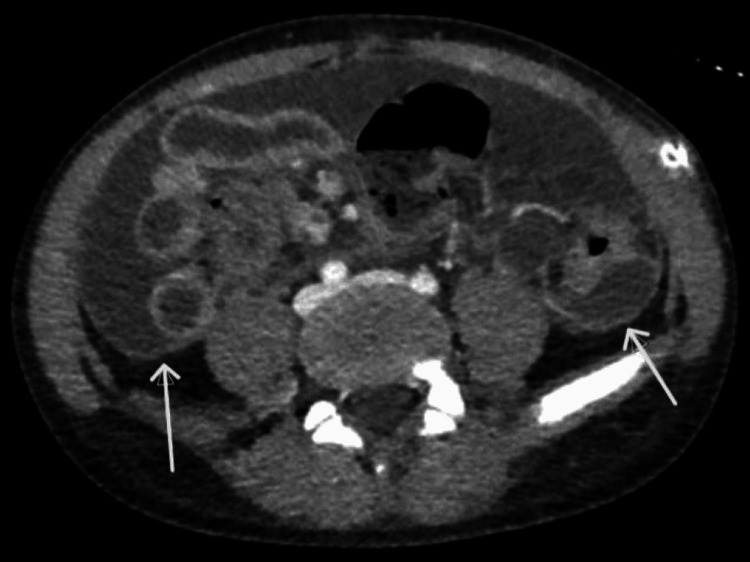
CT abdomen and pelvis (arrows) showing peritoneal wall enhancement with ascites CT: computed tomography
